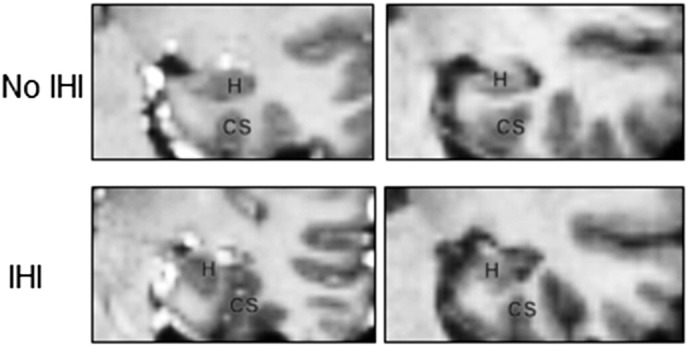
Example of temporal media lobe without and with incomplete hippocampai inversion in MRI coronal view. Top row shows hippocampl (H) without incomplete hippocampal inversion (IHI), they have a flat shape and horizontal orientation and normal sulci shape. Bottom row shows hippocampi (H) with IHI, they have a rounded shape and a medial positioning; the left one presents a deep and vertical collateral sulcus (CS).
